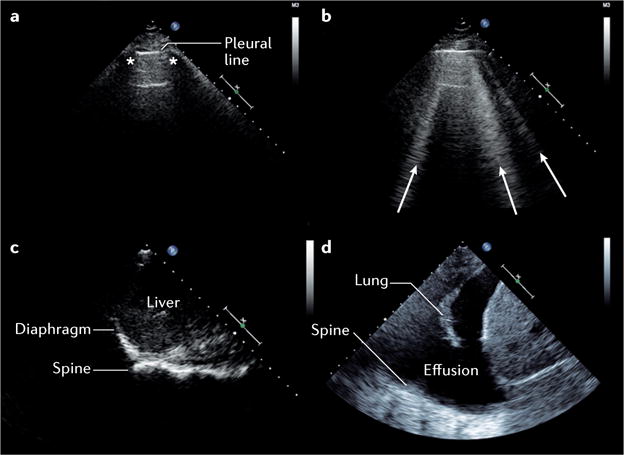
Lung and pleural ultrasonography a | Normal lung with pleural line, and ribs (*) with shadowing. b | Pulmonary oedema with multiple vertical B-lines (arrows) arising from the pleural line. c | Diaphragmatic view with spine ending at the level of the diaphragm, with no pleural effusion. d | Pleural effusion seen as anechoic (echo-free) space above the diaphragm with atelectatic lung. Spine can be visualized beyond the diaphragm owing to the effusion.
